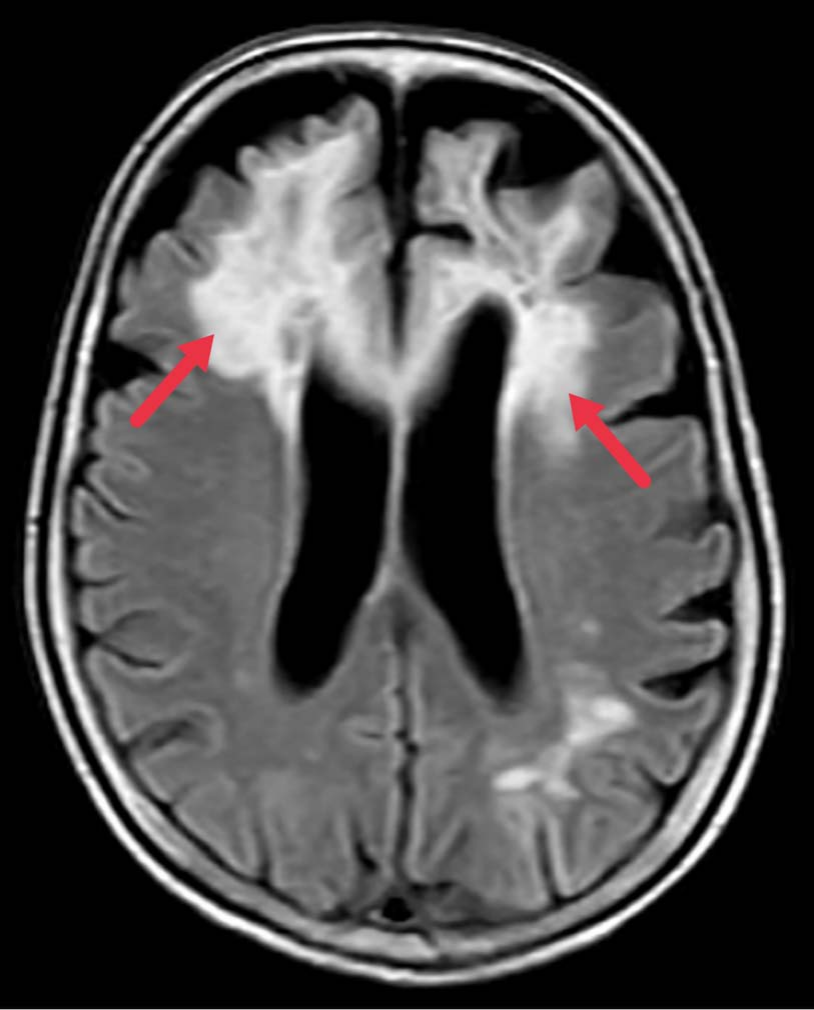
Magnetic resonance imaging head showing periventricular and subcortical white matter changes (red arrows).
